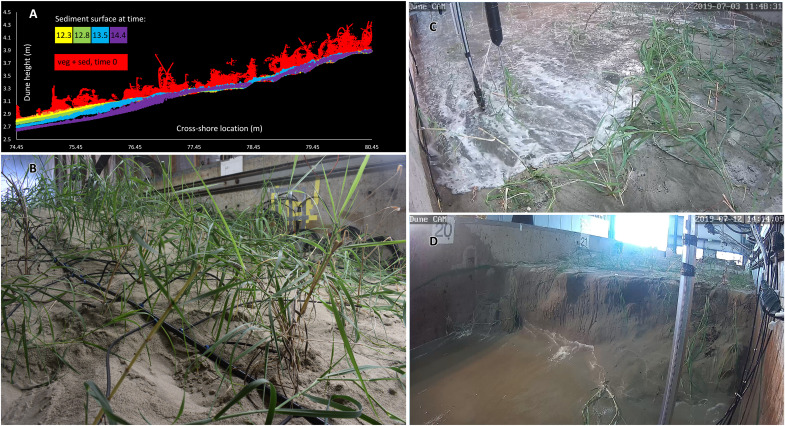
Morphologic changes on VD during the extreme storm event. (A) An example of Terrestrial Laser Scanning (TLS) data for the VD profile, viewed from the cross-shore dimension (i.e., the side), at 0, 12.3, 12.8, 13.5, and 14.4 hours into the storm conditions. The red points depict both the vegetation and the sediment surface before the experiment began at 0 hours. The other colors depict the sediment surface only, between 12.3 and 14.4 hours. By 14.4. hours, an initial 0.1-m tall scarp was apparent at ~76.5 m. (B) *P. amarum* plants on VD before the experiment began at 0 hours. Drip line (black hose) was removed during experimentation. (C) Run-up intercepting plants on VD at 11.3 hours. The depicted sensor array included an optical sediment gauge, sediment movement sensor, ultrasonic sensor, and acoustic velocimeter. (D) A 0.79-m tall scarp on VD at 18.4 hours.
